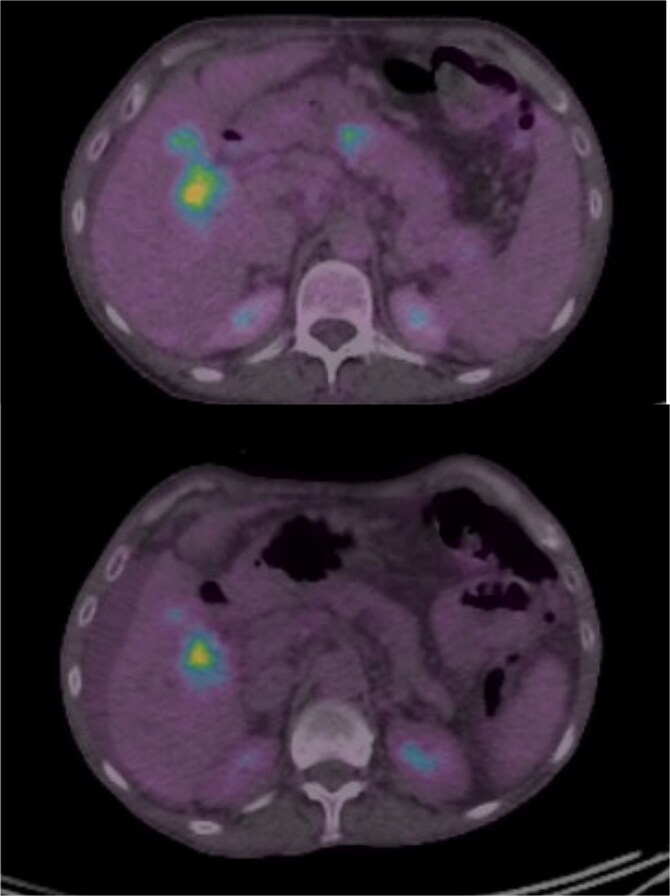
PET-CT scan showing systemic metastatic disease. Metabolic activity in mesenteric, retroperitoneal, and hepatic regions confirming progressive systemic disease.
